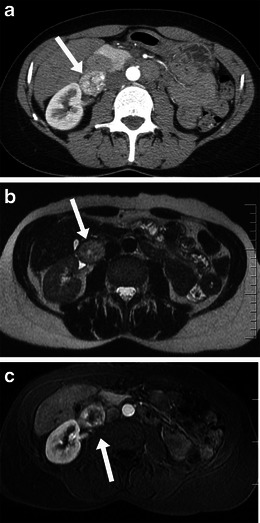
Imaging of paraganglioma. A 43-year-old woman with family history of paraganglioma. Her 24-h urine normetanephrine and norepinephrine levels were elevated. a A well-defined enhancing mass with central necrosis (*arrow*) was seen in the right retroperitoneum. The right adrenal was normal. Axial T2-weighted image (b) shows hyperintense signal (*arrow*), while post gadolinium T1-weighted MRI image (c) shows intense enhancement (*arrow*)
